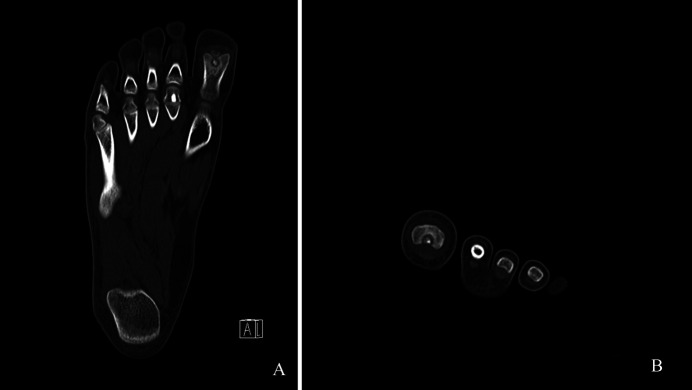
Computed tomography axial (A) and coronal (B) images showing a radiolucent central nidus surrounded by sclerotic rim in the head of the proximal phalanx of the great toe.
